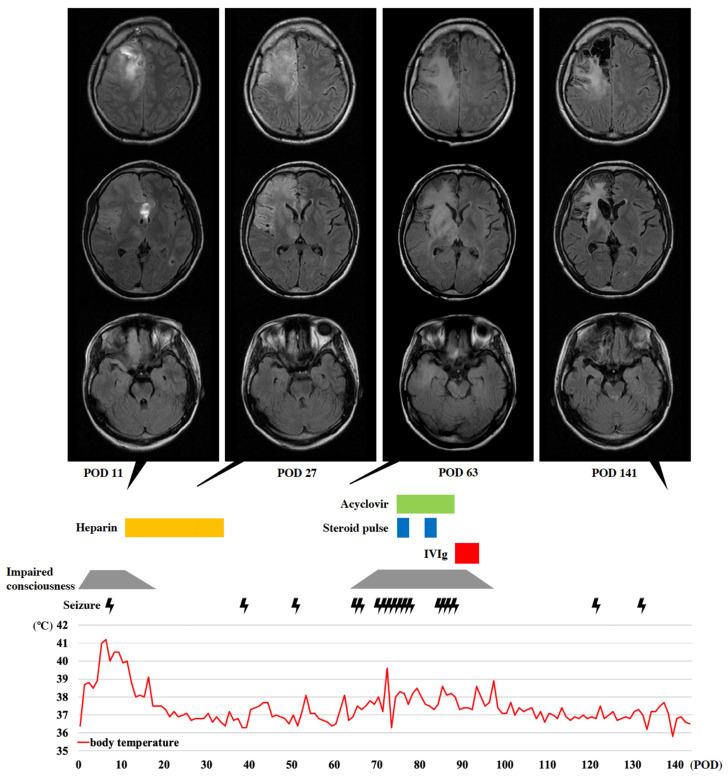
The course of clinical and MRI findings in the patient with anti-NMDAR encephalitis after CC. From POD 1, she showed fever, impaired consciousness, and left hemiplegia. On POD 11, MRI revealed a right frontal cerebral hemorrhage and right dominant bilateral frontal lobe edema with midline shift. This hyperintensity on FLAIR appeared predominantly in gray matter and also in the right orbitofrontal lobe and thalamus, but not in the pyramidal tract. Heparin therapy for CVST dramatically improved the impaired consciousness, fever, and MRI findings, such as cerebral edema and midline shift (POD 27). However, the FLAIR-hyperintense area expanded to the right precentral gyrus, basal ganglia, posterior limb of the internal capsule, and temporal lobe (POD 63). Around this time, she started to experience impaired consciousness, fever, and cluster seizure, which gradually worsened. Although steroid pulse and intravenous acyclovir did not improve these symptoms, IVIg therapy immediately improved them and reduced the FLAIR-hyperintense area (POD 141).
